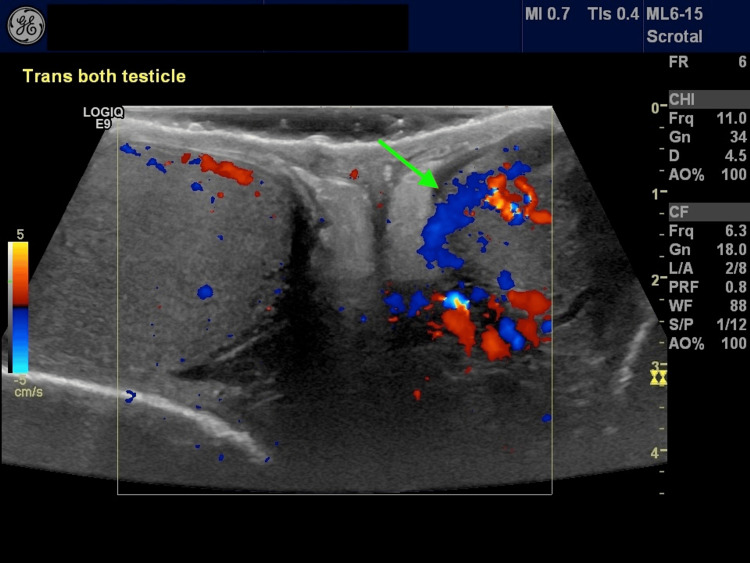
Testicular ultrasound showing marked hyperemic left epididymis consistent with epididymitis (green arrow)
